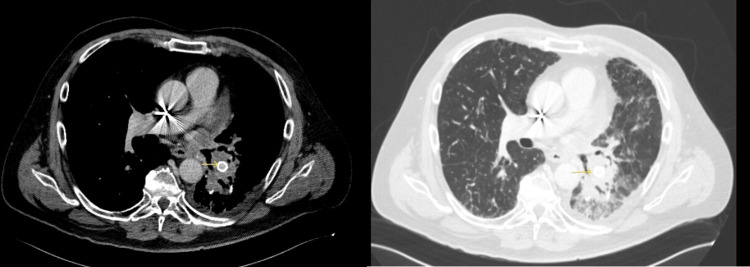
Chest CT Tumor involvement at the left secondary carina, proximity with the inferior pulmonary artery with the stent in place.
